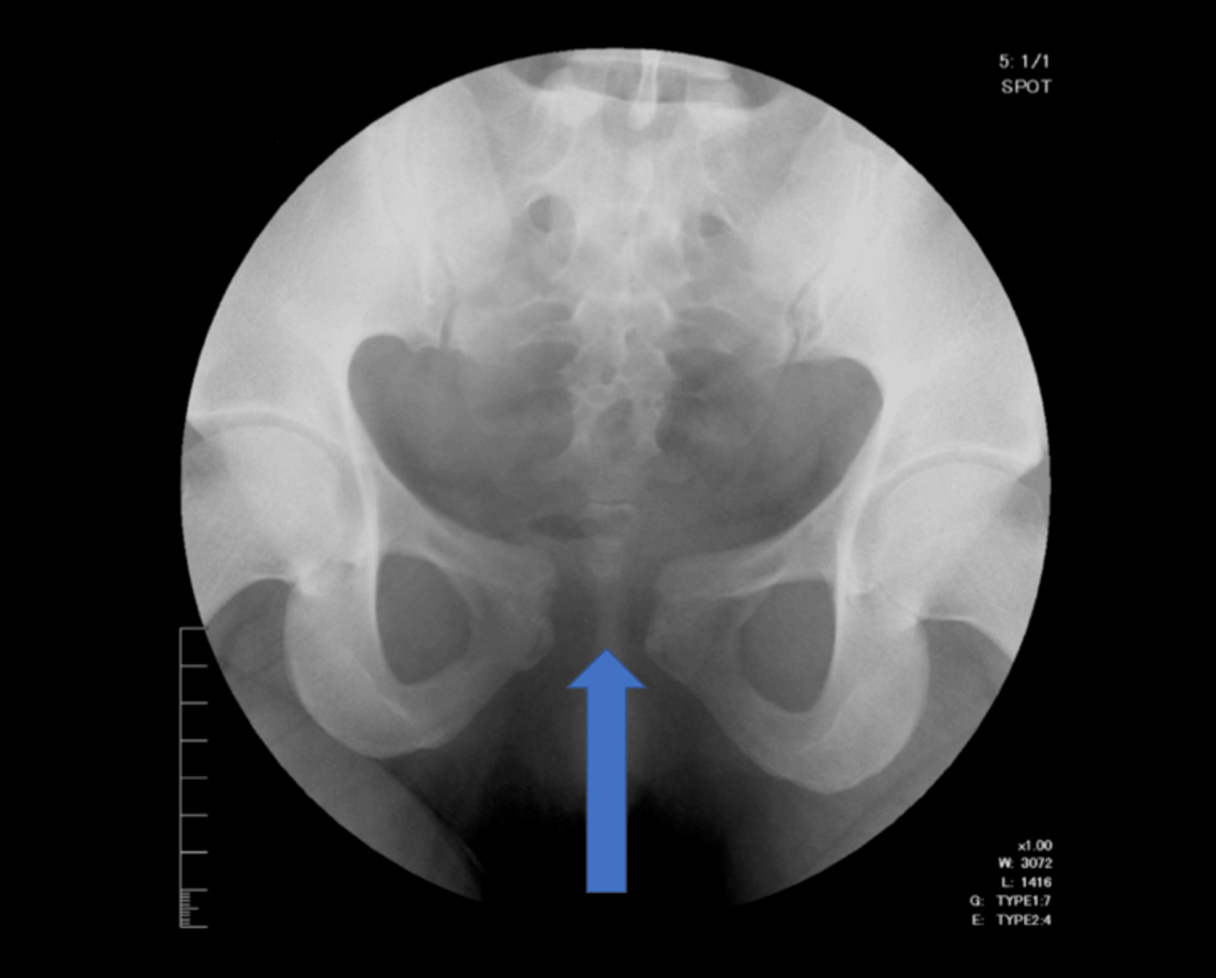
Anteroposterior radiograph of the pelvis showing pubic symphysis diastasis Anteroposterior pelvic radiograph of a 22-year-old female with ADPKD. The arrow indicates widening of the pubic symphysis measuring 25.5 mm. No sacroiliac joint asymmetry, fractures, infection, or degenerative changes are present, consistent with idiopathic pubic symphysis diastasis.
